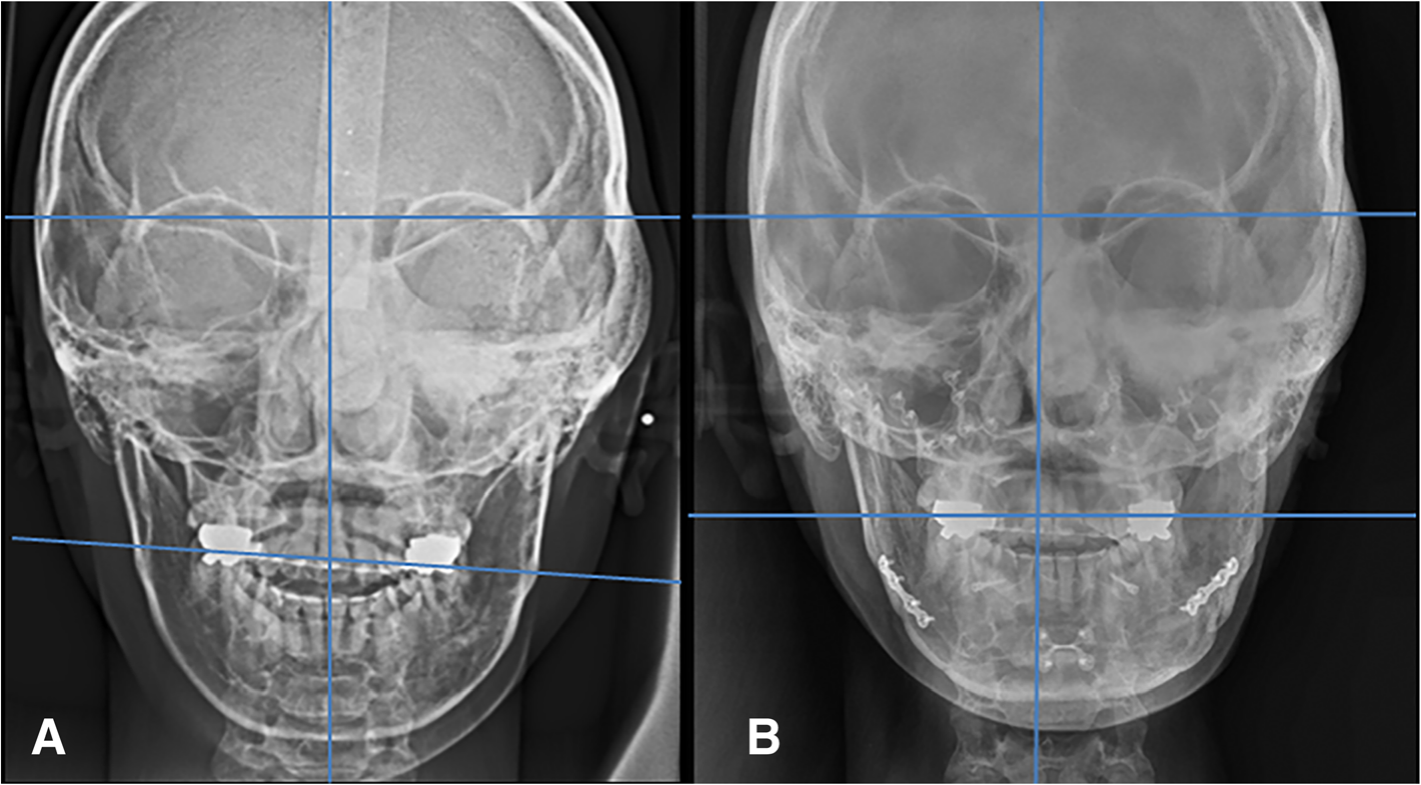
Preoperative (a) and 2 years postoperative radiographic image (b) of patient no. 2
